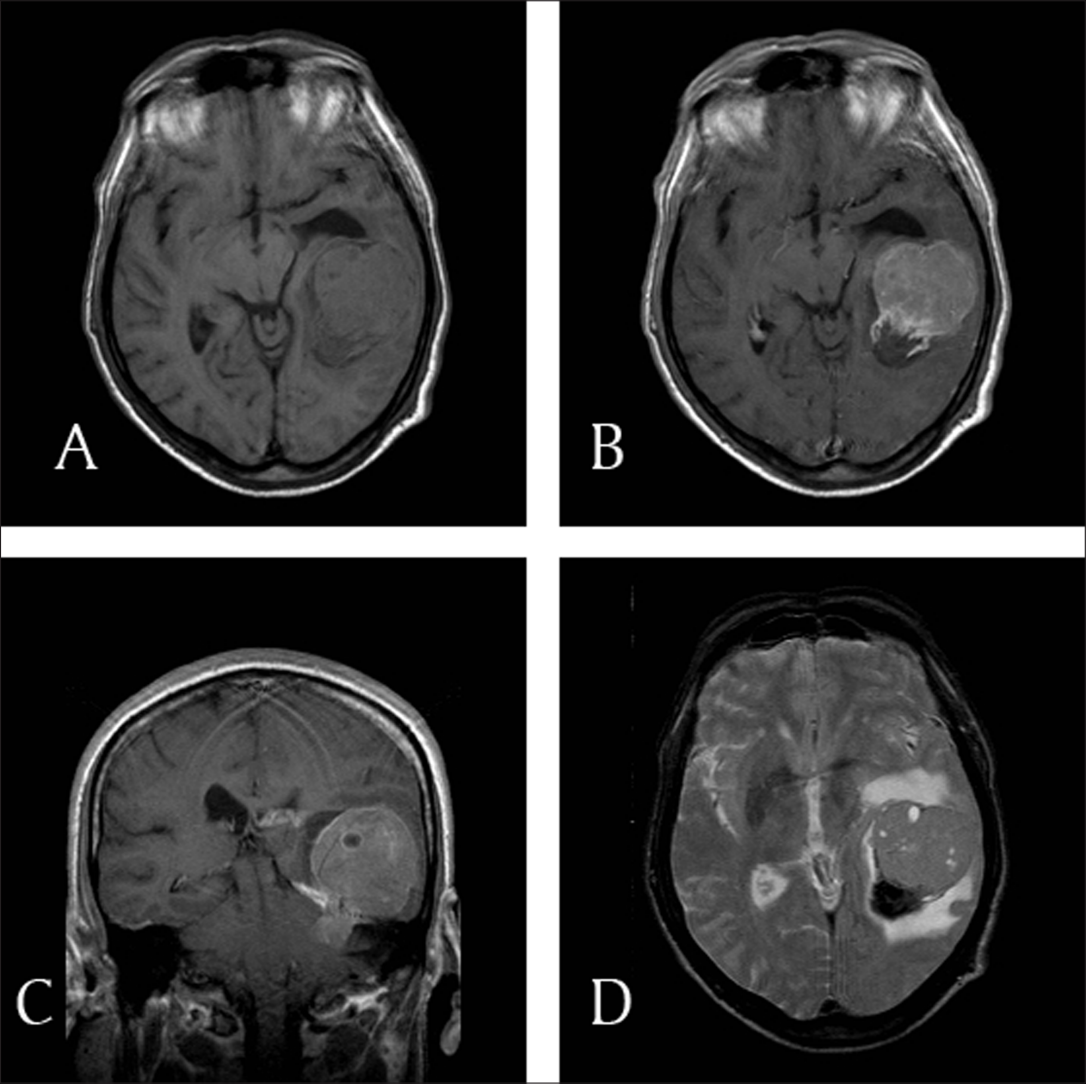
(A) Axial unenhanced T1-weighted image: The lesion appears isointense to grey matter image. There are no spontaneously hyperintense areas within the lesion. Dilatation of the temporal horn of the left lateral ventricle is evident. (B) Gadolinium enhanced T1-weighted image: Moderate, heterogeneous contrast enhancement which is more pronounced at the periphery. There is no enhancement posteriorly in the lesion. (C) Coronal gadolinium enhanced T1-weighted image: Rim enhancement surrounding the cystic areas can also be seen on this coronal image. A small component of the tumor extends below the thickened tenorium cerebelli in the left cerebellopontine angle. The tumor is involving the supra- and infratentorial surface of the tentorium and therefore seems to be dural based. (D) Axial T2-weighted image: This image is slightly more cranial than images (A) and (B) and reveals a lesion that is isointense with grey matter, with multiple cystic/necrotic areas and surrounding vasogenic edema. The posterior part of the tumor contains hypointense blood degradation products.
